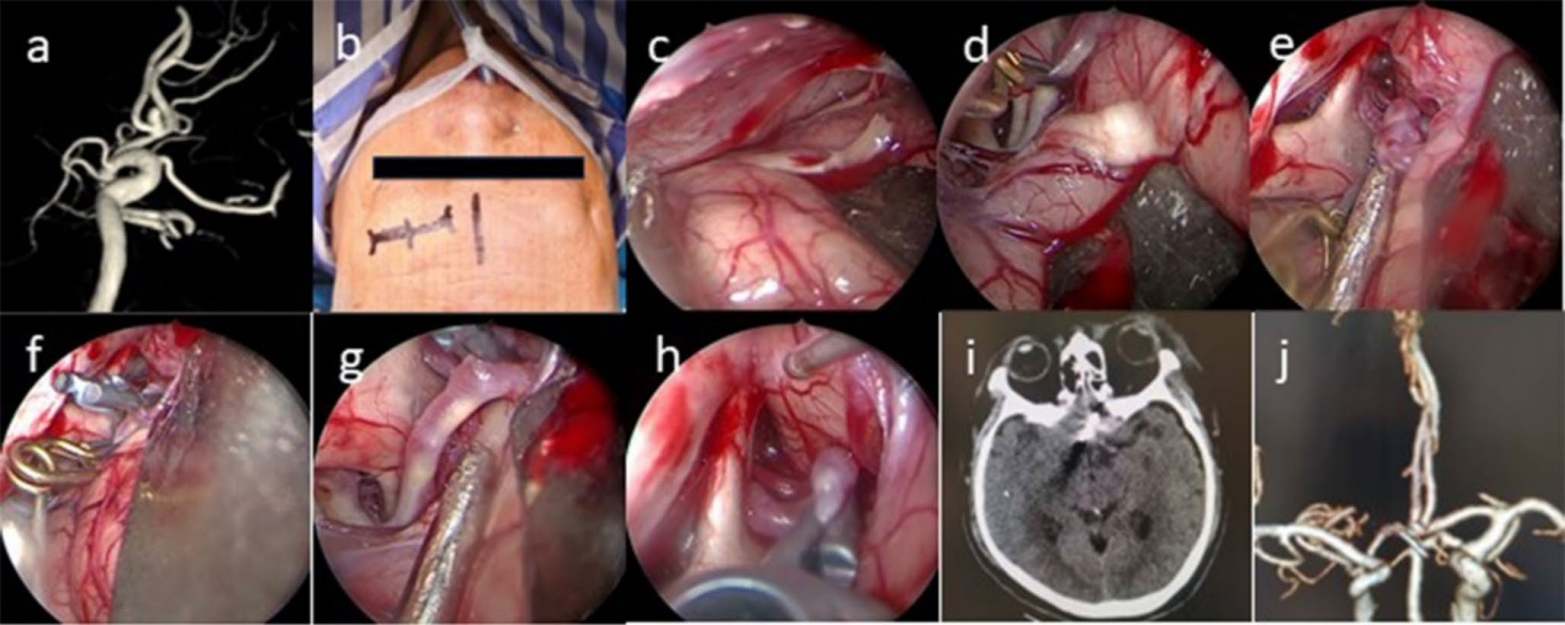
(a) Preoperative DSA confirmed the diagnosis of an ACoA aneurysm. (b) Due to the patient’s dominant left A1, the left SKA approach was chosen. (c) The arachnoid membrane at the base of the anterior cranial fossa was carefully opened, allowing for the release of CSF. (d) A temporary occlusion of the A1 segment was performed to facilitate the procedure. (e) Distal exploration led to the identification of the aneurysm. (f) The aneurysm was successfully clipped. (g) Following the clipping, the temporary clip was removed. (h) The parent artery and its branches were meticulously examined to ensure proper blood flow. (i) Postoperative CT scan showing no bleeding or ischemic changes. (j) Postoperative CTA scan showed that aneurysm was clipped completely and the parent artery and its branches were preserved
